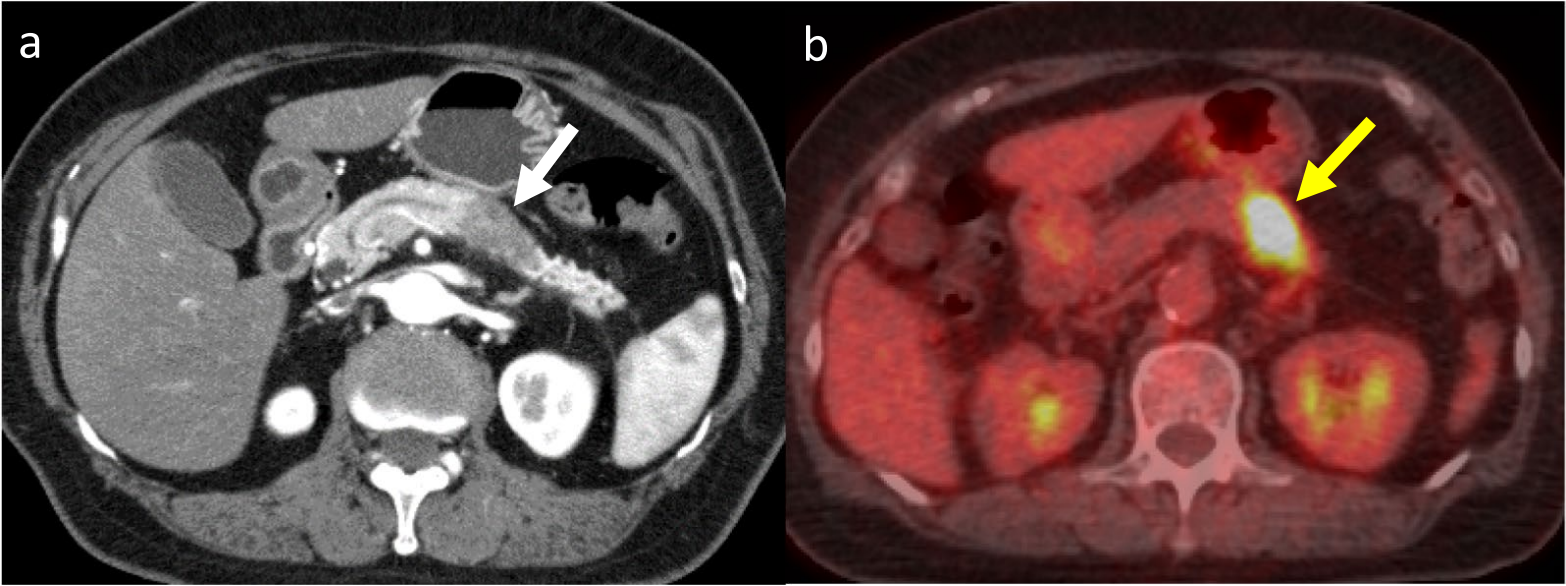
Imaging results at admission. a Contrast-enhanced abdominal computed tomography showed a 35-mm hypovascular mass in the tail of the pancreas (white arrow). b
